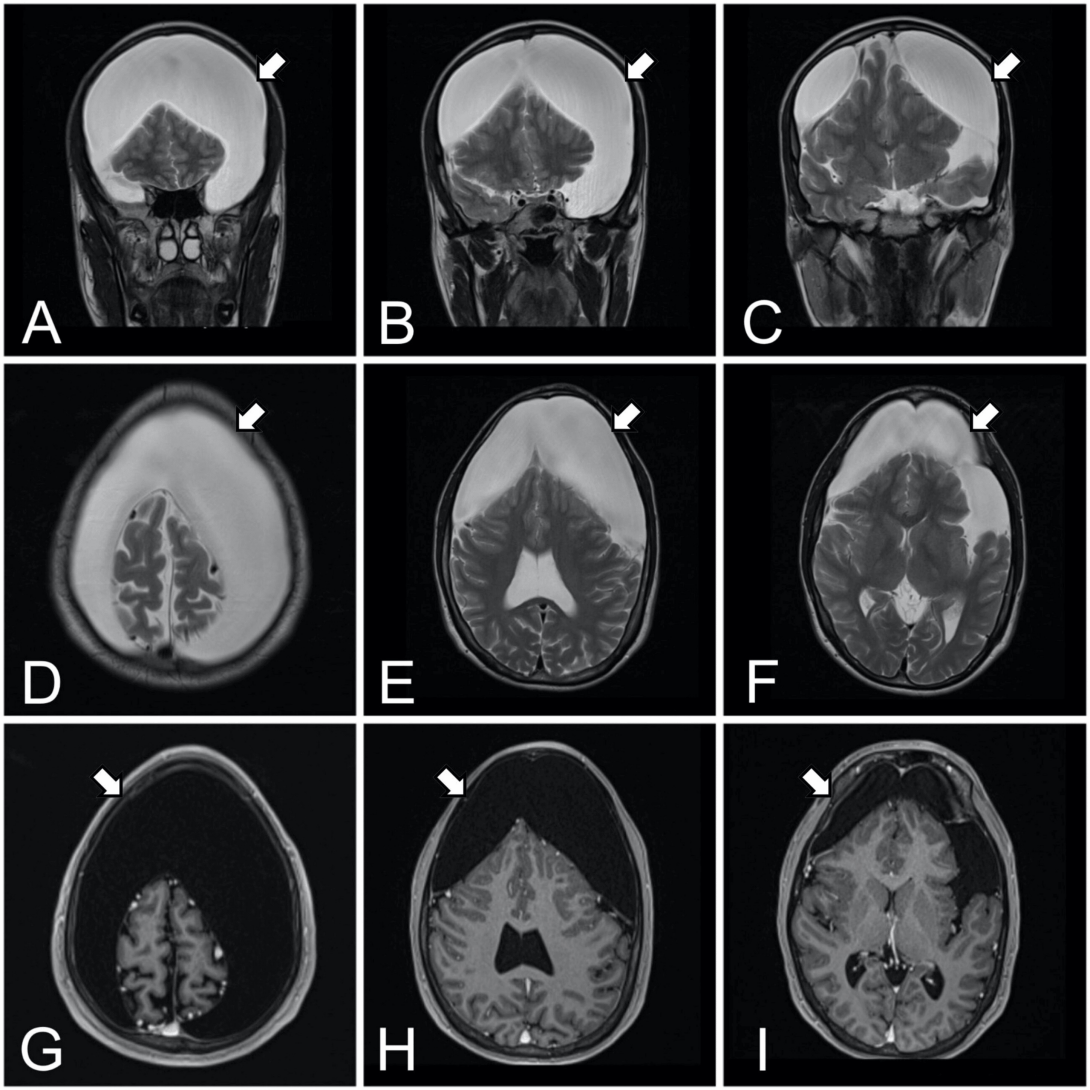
Brain Magnetic Resonance Imaging (MRI) Simple cranial MRI, T2-weighted coronal slices (A-C), T2-weighted axial slices (D-F). Contrast MRI axial slices in T1 weighting (G-I). The presence of arachnoid cysts occupying the region of the frontal lobes, hyperintense in T2, hypointense in T1, and without enhancement following contrast administration. The remaining cerebral hemispheres showed no evidence of space-occupying lesions, with normal convolution depth and well-differentiated sulci and fissures. Additionally, the cerebellum, cerebellar base, pons, and medulla were unremarkable with no abnormalities observed.
